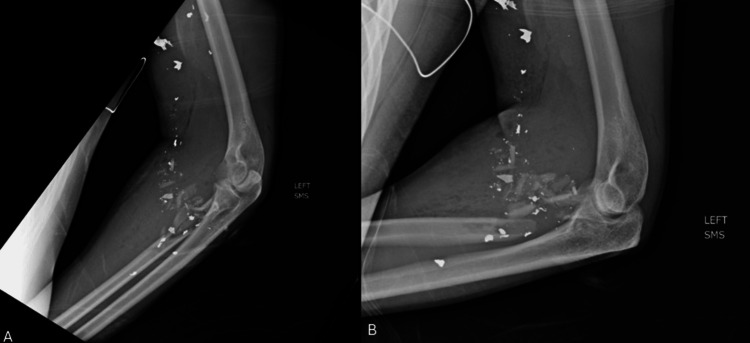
Injury radiographs Anteroposterior (A) and lateral (B) radiographs of the left elbow demonstrating a comminuted proximal radial shaft fracture from a ballistic injury.
